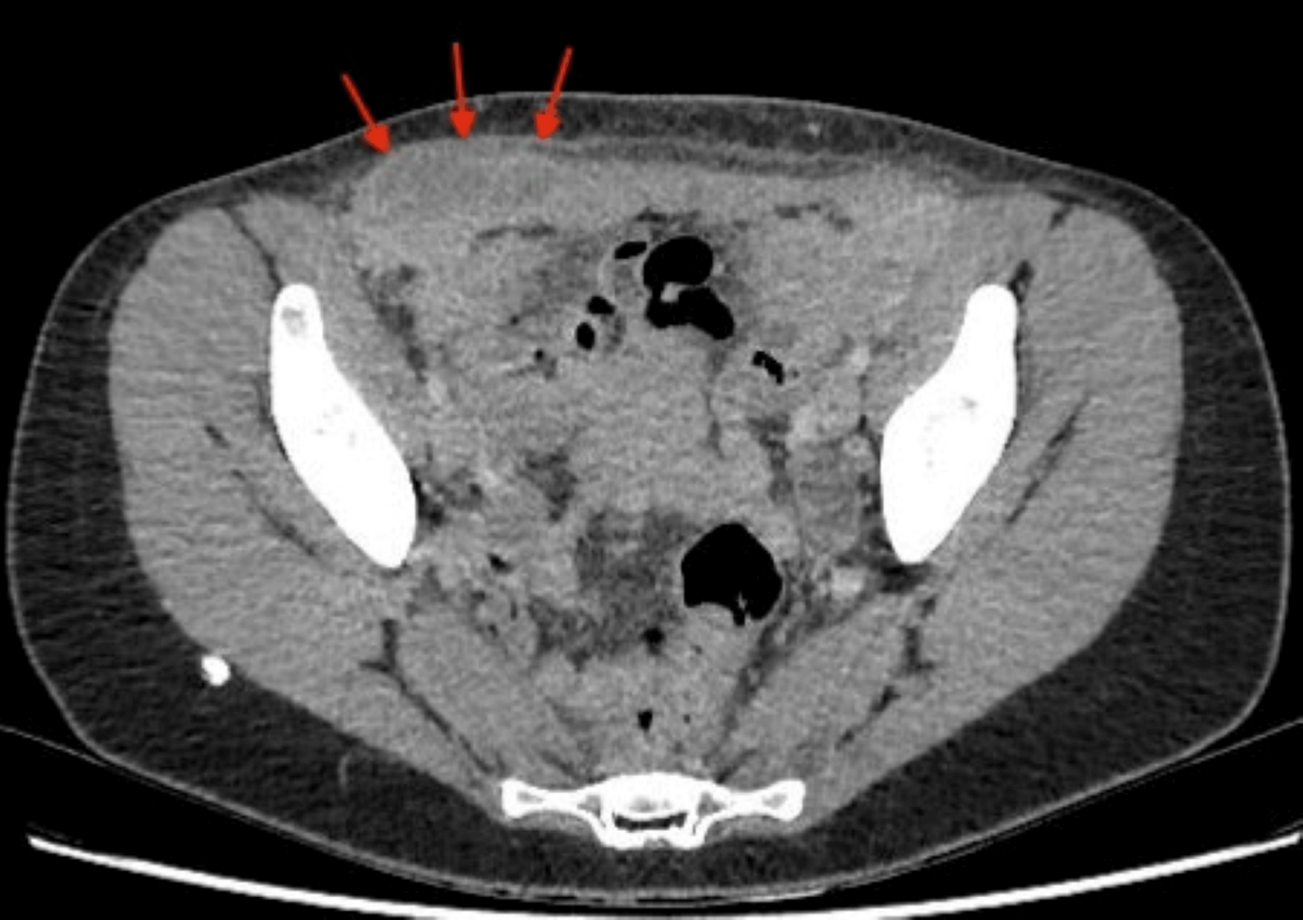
Axial image of CT abdomen showing a nonspecific inflammation in the peritoneal cavity.
